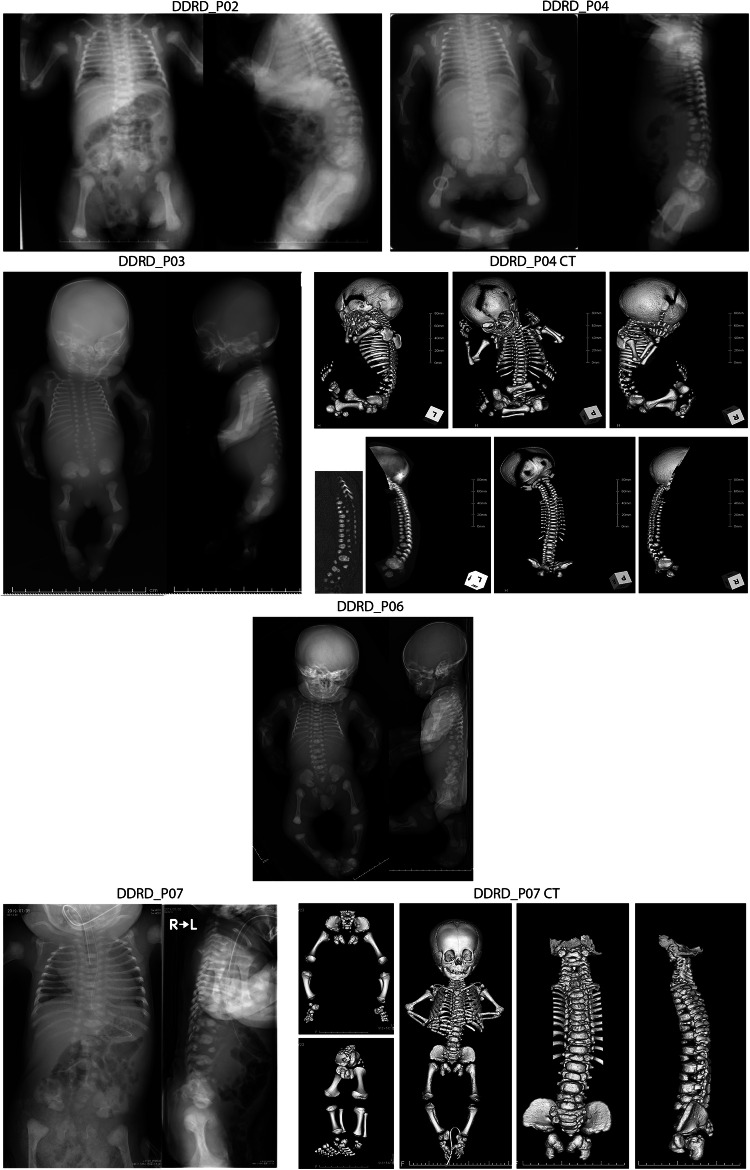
Representative X-ray images and reconstructed CT images of DDRD_P02, P03, P04, P06, and P07
